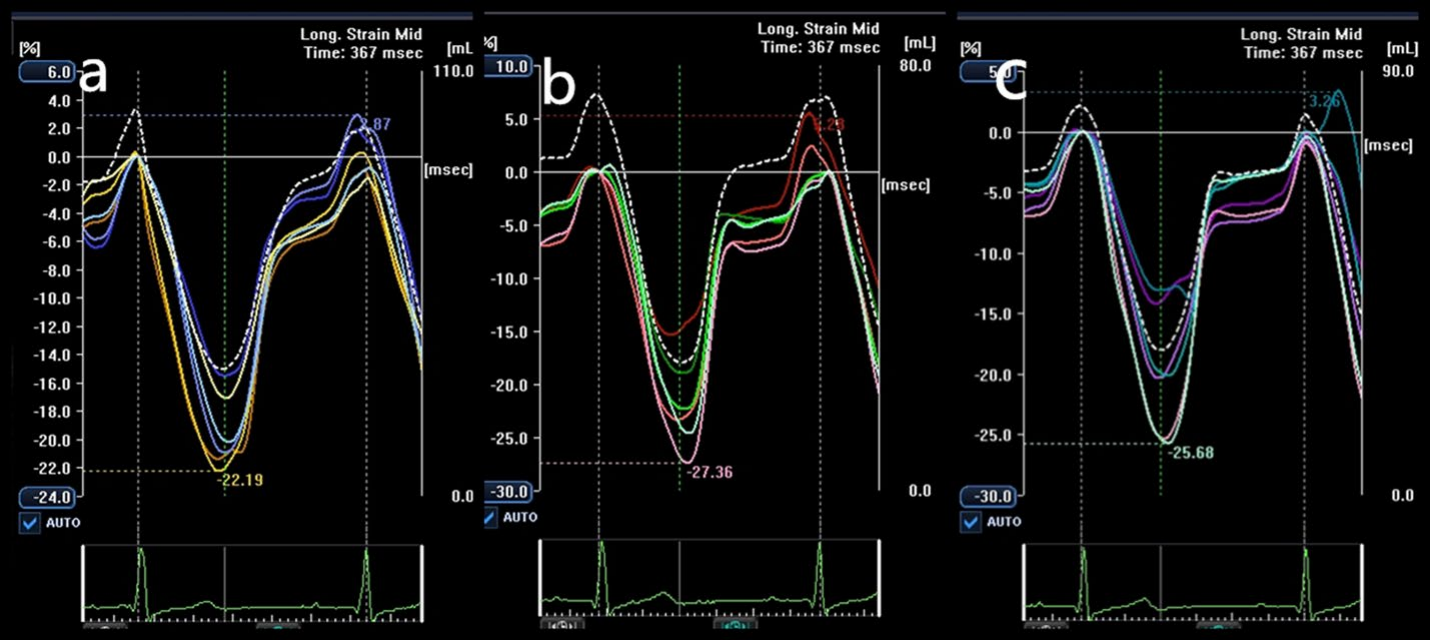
Example of 2DSTE time-longitudinal strain curves. a–c represent the apical 2-, 3- and 4-chamber view, respectively. The different color curves represent corresponding regional segments
